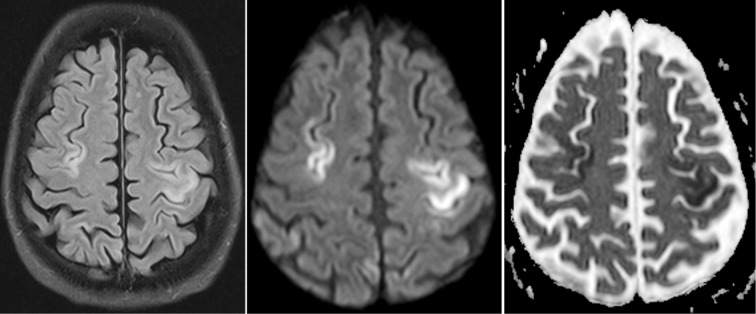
30 year SLE pt. (aPL) with left hemiparesis. Axial FLAIR (left), diffision weighted (middle) and ADC (right) images show multiple foci of diffusion restriction representing acute infarcts in bilateral parietal lobes.
